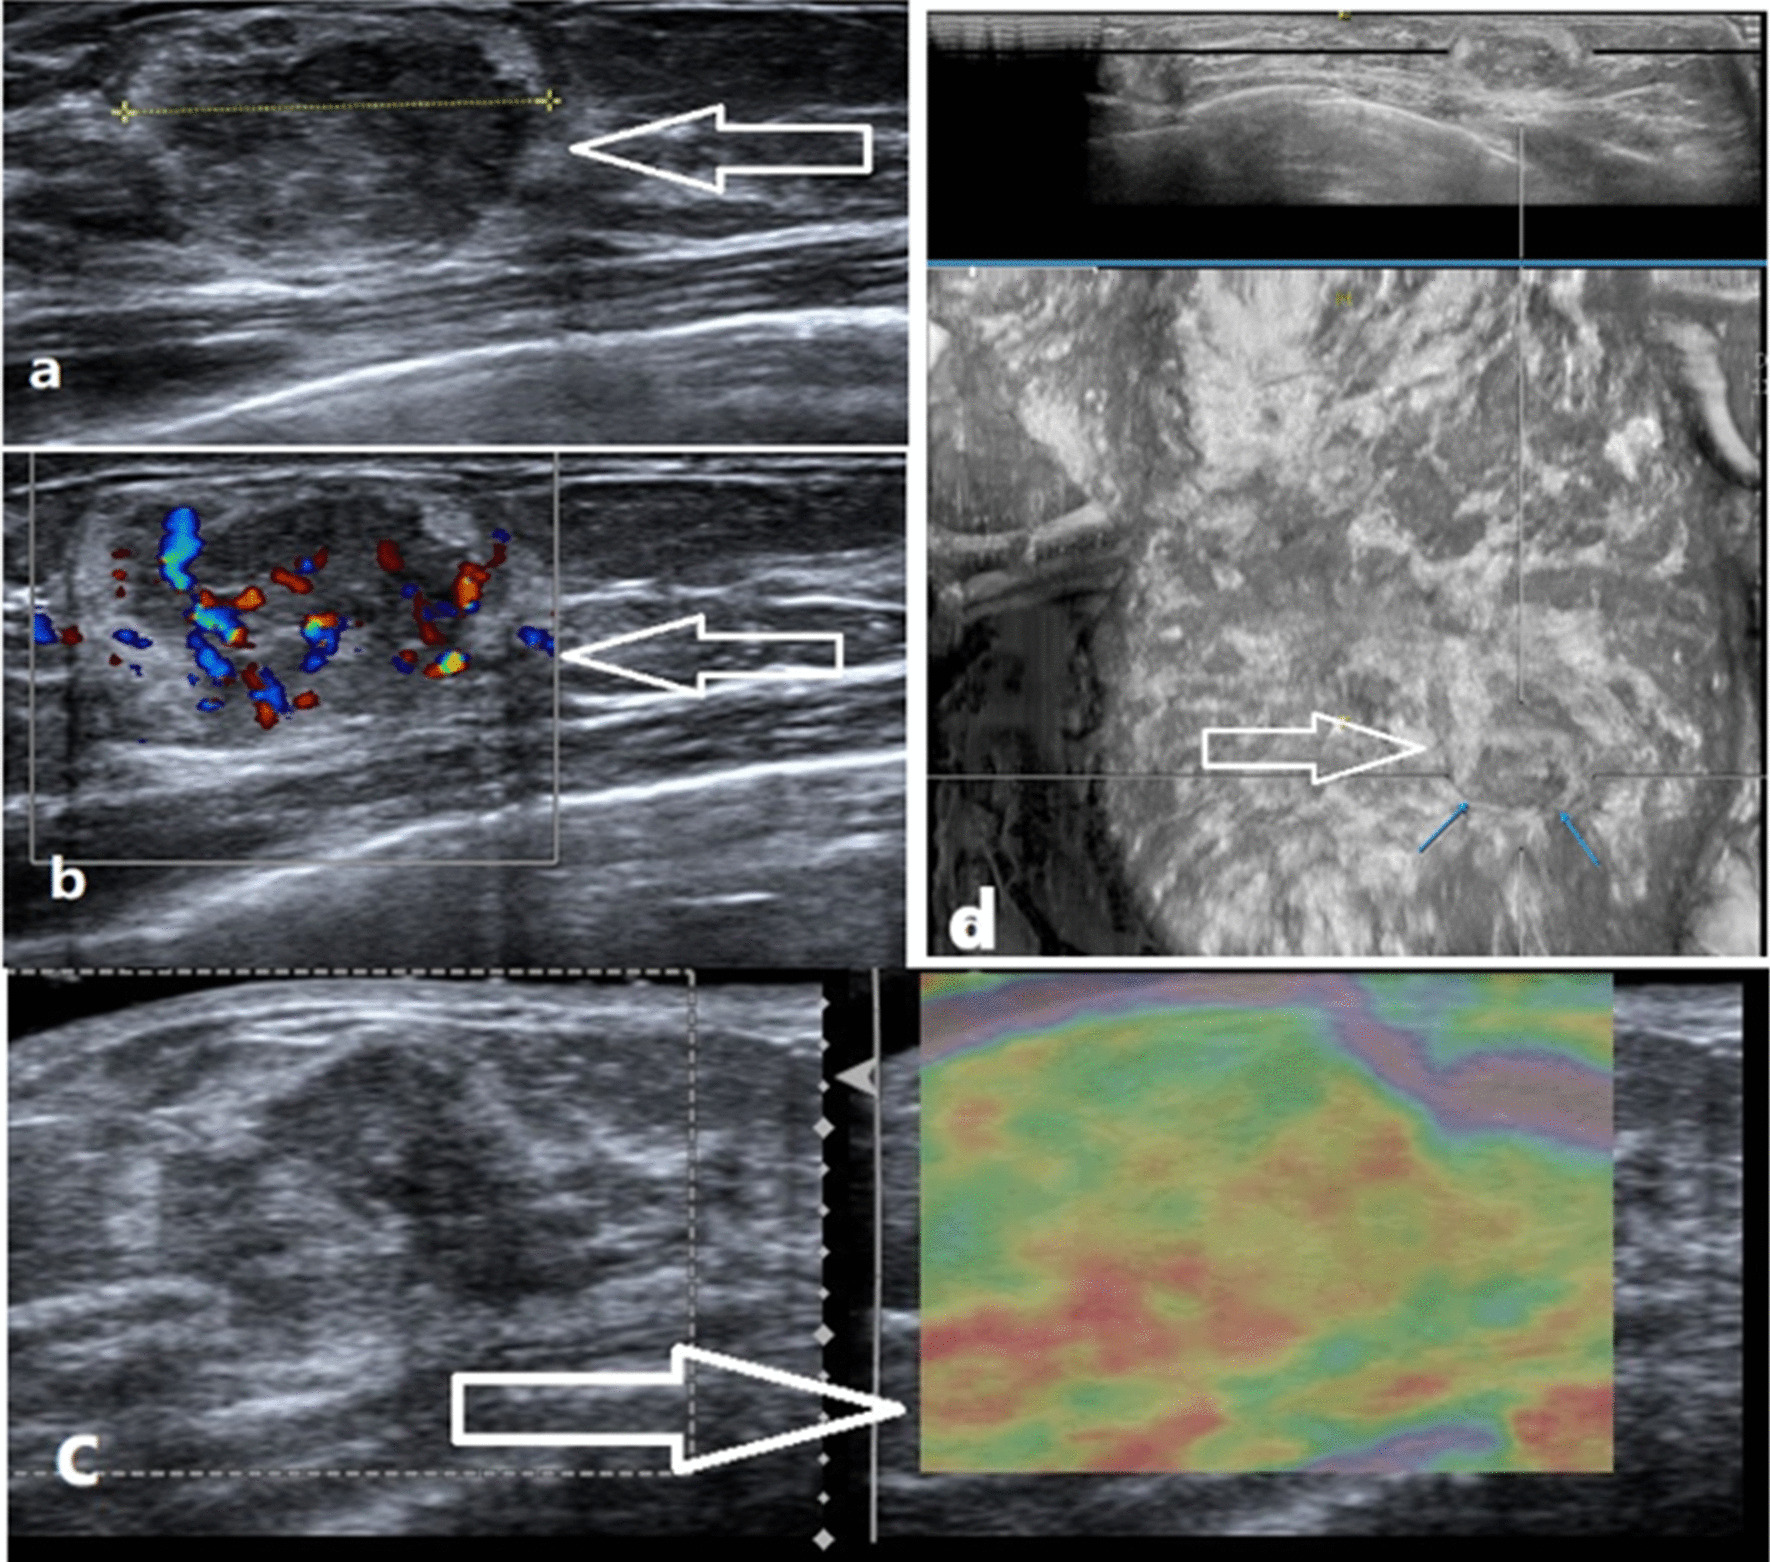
a The hand-held ultrasound revealed an irregular and uneven 22 × 13 × 21 mm hypoechoic mass at 3 o 'clock in the right breast with an echo halo extending from the anterior edge of the breast area to the peribreast adipose layer (white arrow). b Color Doppler imaging showed some vascular distribution in the mass(white arrows). c Ultrasound elastography showed a Tsukuba stiffness score of 3 of the lesion (white arrows). d By ABUS, the lesion showed an irregular shape, an indistinct margin, heterogeneous hypoechoic with an echogenic halo (white arrows)
